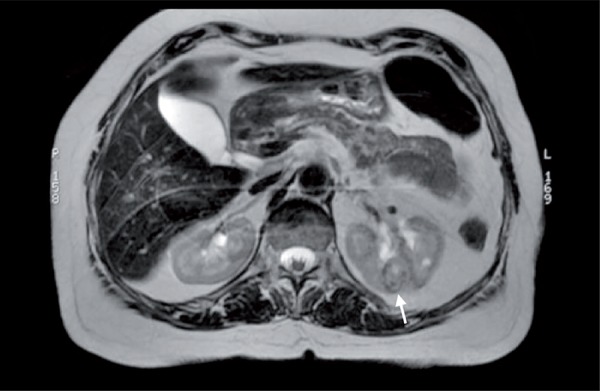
MR image, T2 sequence. Arrow: A biopsy-proven 2.2 cm clear cell carcinoma on the left kidney.
